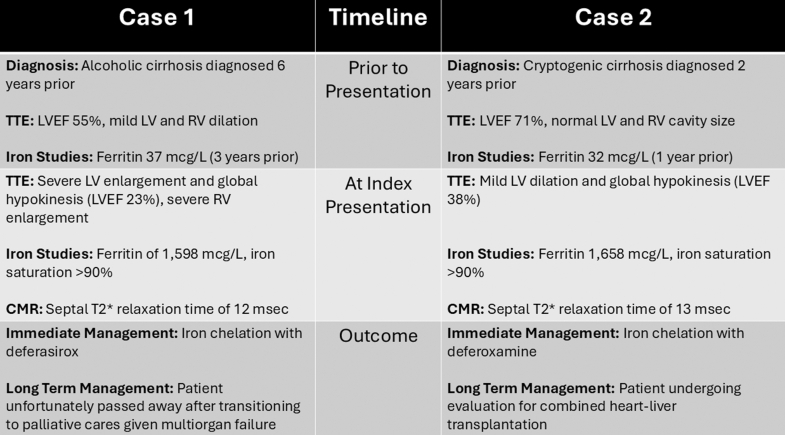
Clinical Course, Laboratory, and Imaging Findings of Patients With Iron Overload Cardiomyopathy Secondary to Cirrhosis CMR = cardiac magnetic resonance imaging; LV = left ventricular; LVEF = left ventricular ejection fraction; RV = right ventricular; TTE = transthoracic echocardiogram.
